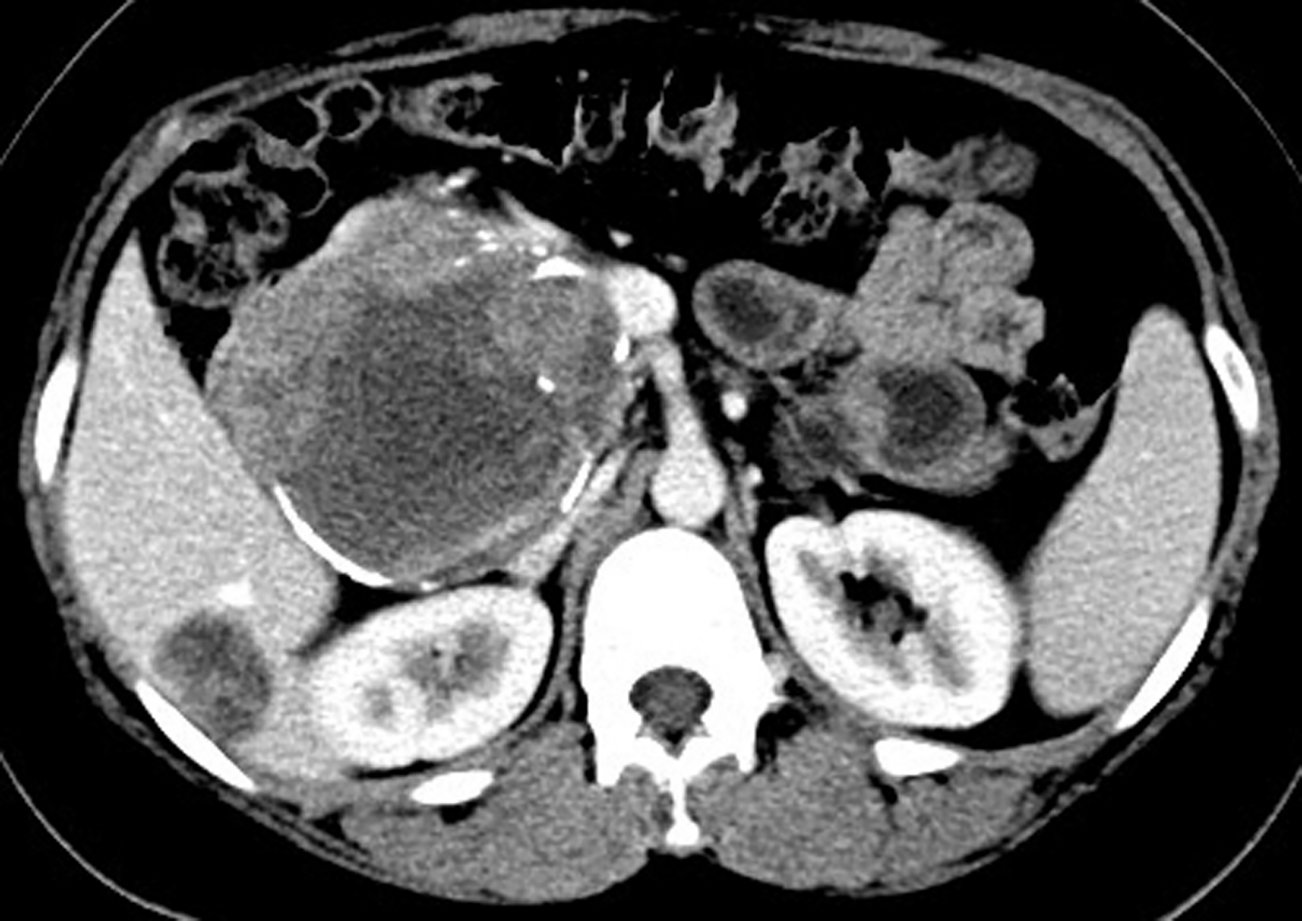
Abdominal CT showing SPT located in head of pancreas and liver metastatic lesion in the right lobe.
